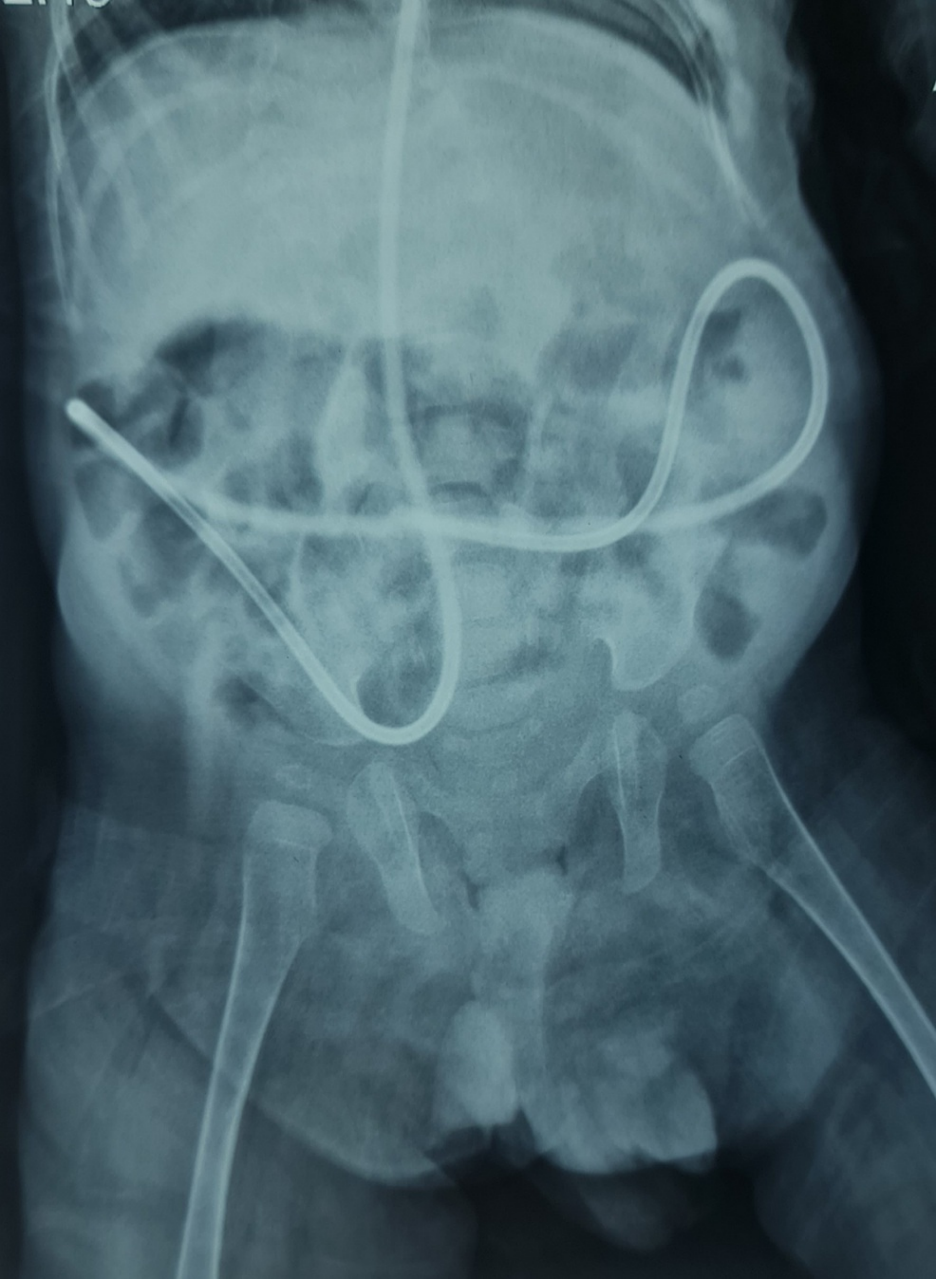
X-ray abdomen showing VP shunt successfully reduced back into the peritoneum.
